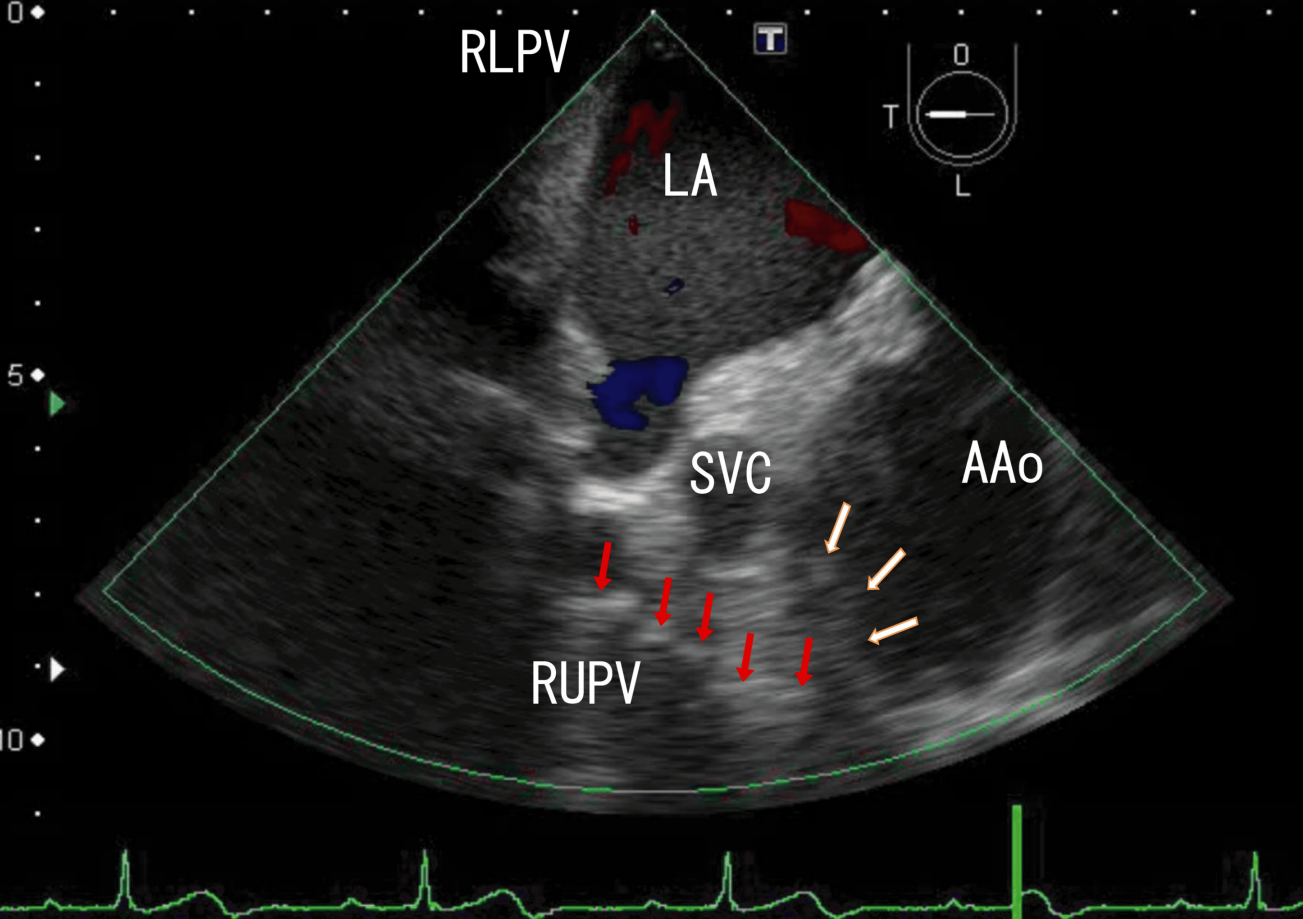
TEE images showing decreased AAo thrombi and approaching several white parts TEE revealed large thrombi in the AAo (white arrows), and a series of white parts remained, which were directed to the RUPV (red arrows). The blood flow in the AAo increased, indicating that the AAo thrombi were partially resolved. Blood flow from the RUPV and the RMPV did not change, indicating that the LA thrombi did not resolve, as shown in dark white. The LA thrombi from the RLPV disappeared. TEE: transesophageal echocardiography, AAo: ascending aorta, LA: left atrium, SVC: superior vena cava, RLPV: right lower pulmonary vein, RUPV: right upper pulmonary vein, RMPV: right middle pulmonary vein
